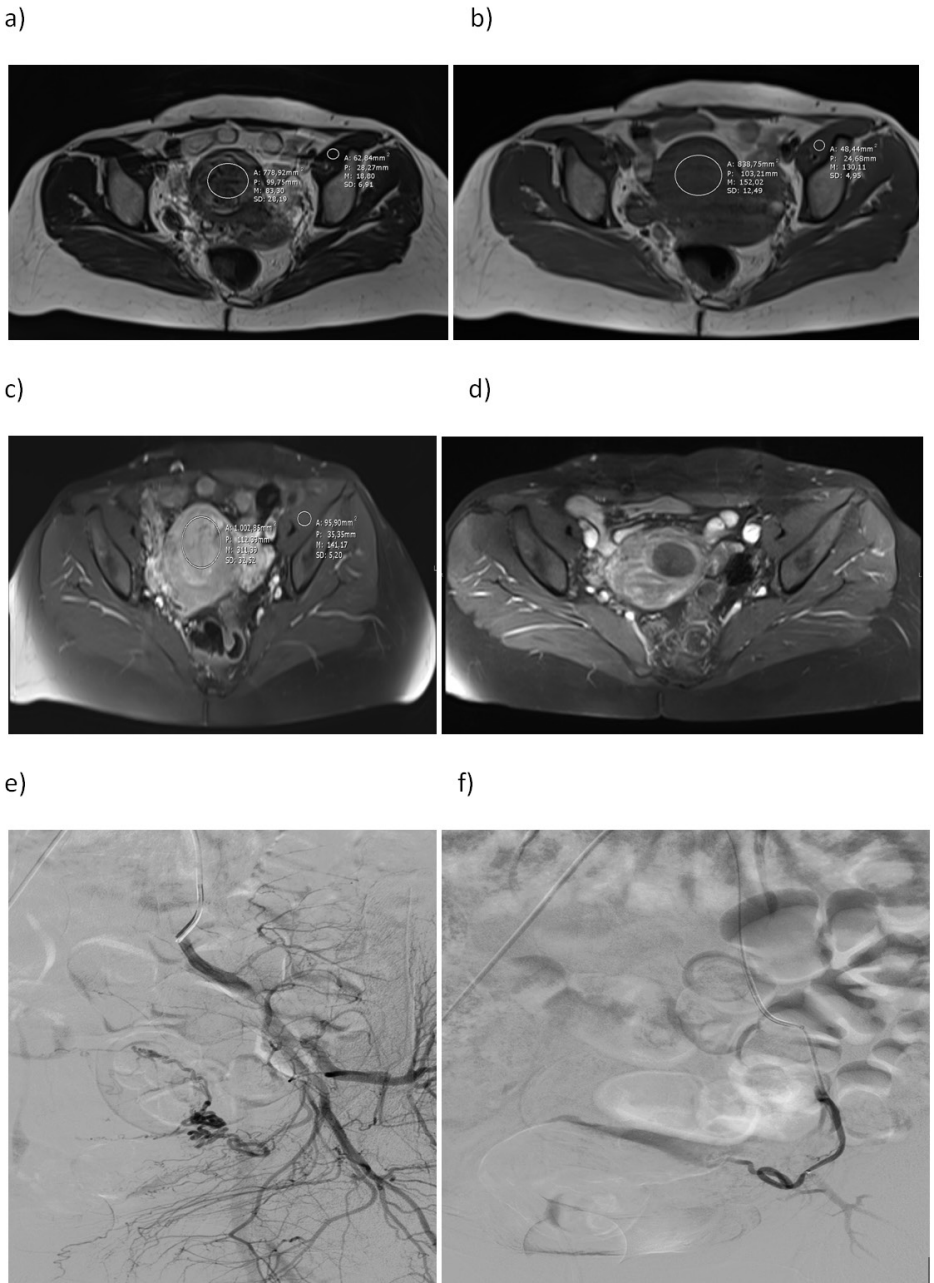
In the example case, SI ratios calculated before the embolization procedure were 4.61 in T2W, 1.16 in T1W, and 2.1 in post-contrast T1W MR images. Myoma volume was 45 cc before UAE and regressed to 10 cc after the procedure (ad). In addition, Figures e-f show DSA images obtained selectively from the left uterine artery before and after embolization.
